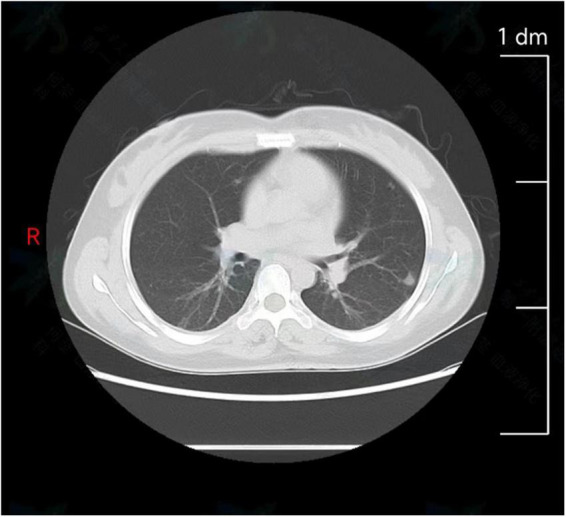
Chest computed tomography (CT) revealed multiple pulmonary nodules in both lungs.
